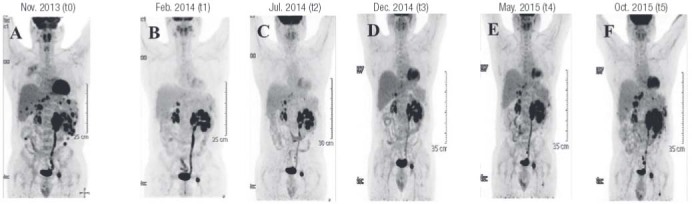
18FDG-PET before (A) and during (B-F) sunitinib therapy.
